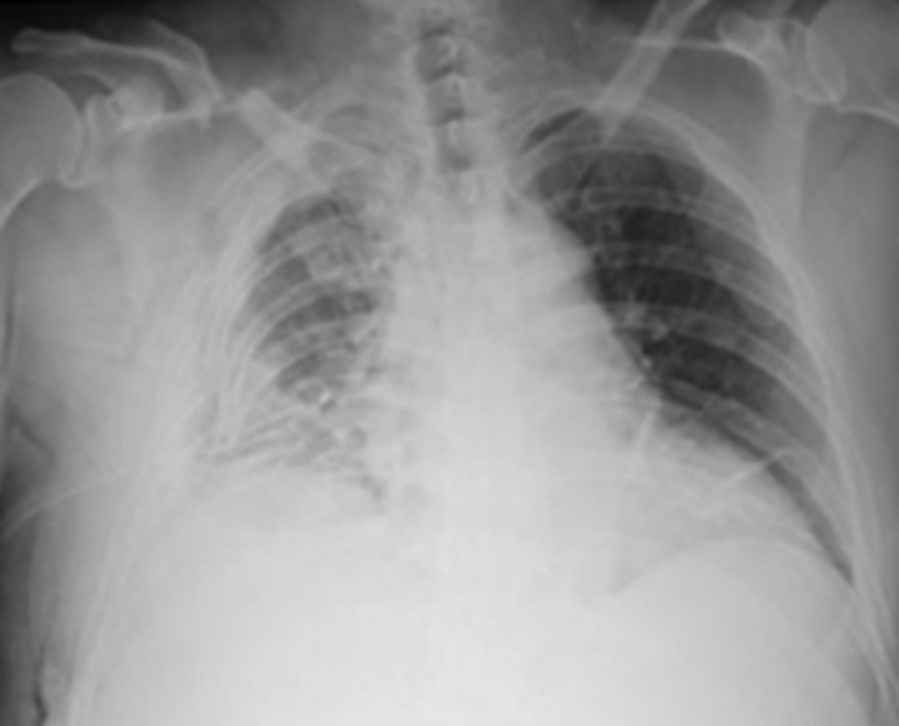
Chest X-ray showed advanced chest wall destruction with rib fractures and a collapsed lung
